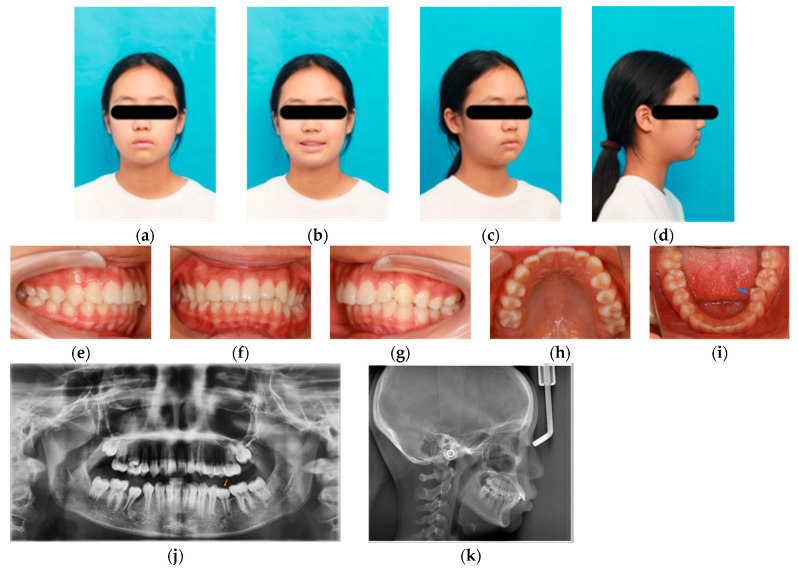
Facial, panoramic, and intraoral photographs obtained at the 2-year follow-up after treatment. (a–d) Facial photographs at the 2-year follow-up. (e–i) Intraoral photographs at the 2-year follow-up, showing eruption of tooth 35 to the occlusal plane with mesial rotation and slight lingual inclination; tooth 35 is indicated by the blue arrow in figure (i). (j) Panoramic radiograph at the 2-year follow-up; tooth 35 is indicated by the orange arrow. (k) Lateral cephalometric radiograph at the 2-year follow-up.
